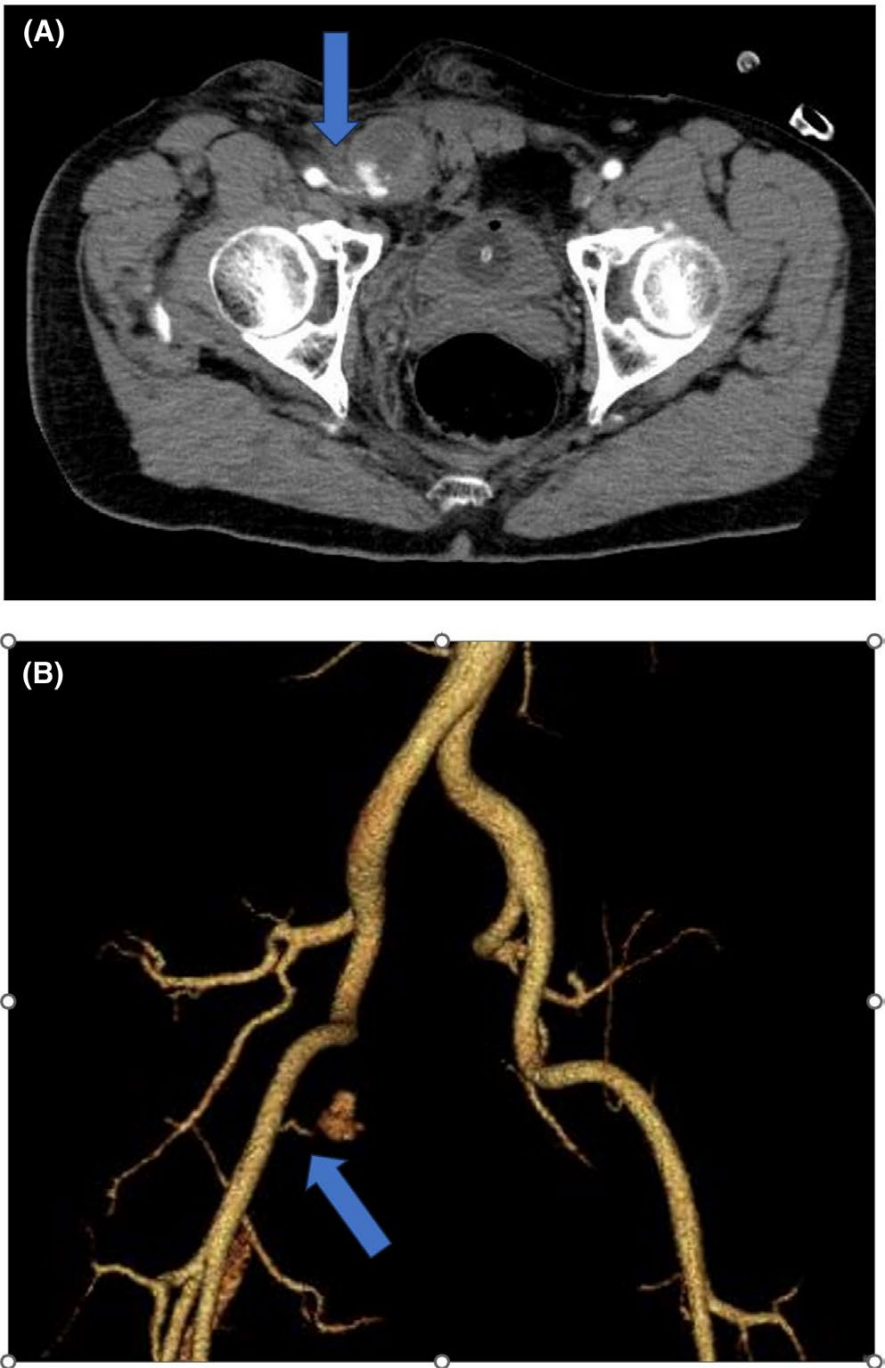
Contrast‐enhanced CT scan after manual compression. (A) The blue arrow indicates contrast leakage from the right femoral artery into the right inguinal canal. (B) The blue arrow indicates bleeding from a branch of the right femoral artery.
